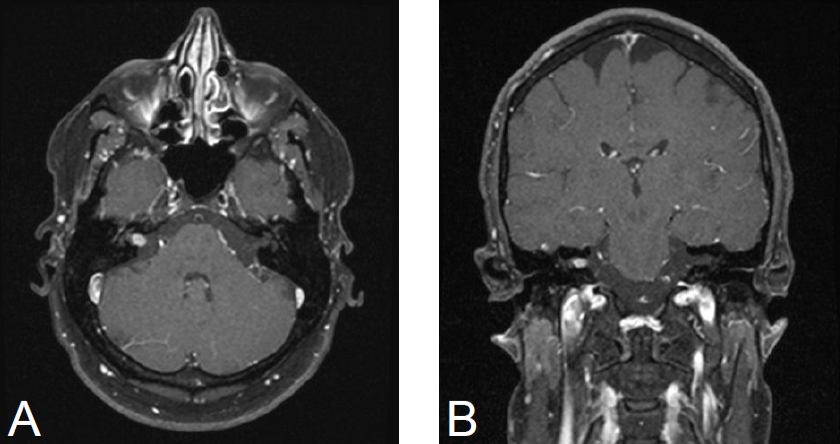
MRI, T1 FS + CM; A axila; B coronal. Intra- und slightly extrameatal vestibular on the right measuring 9x5x5 mm.
